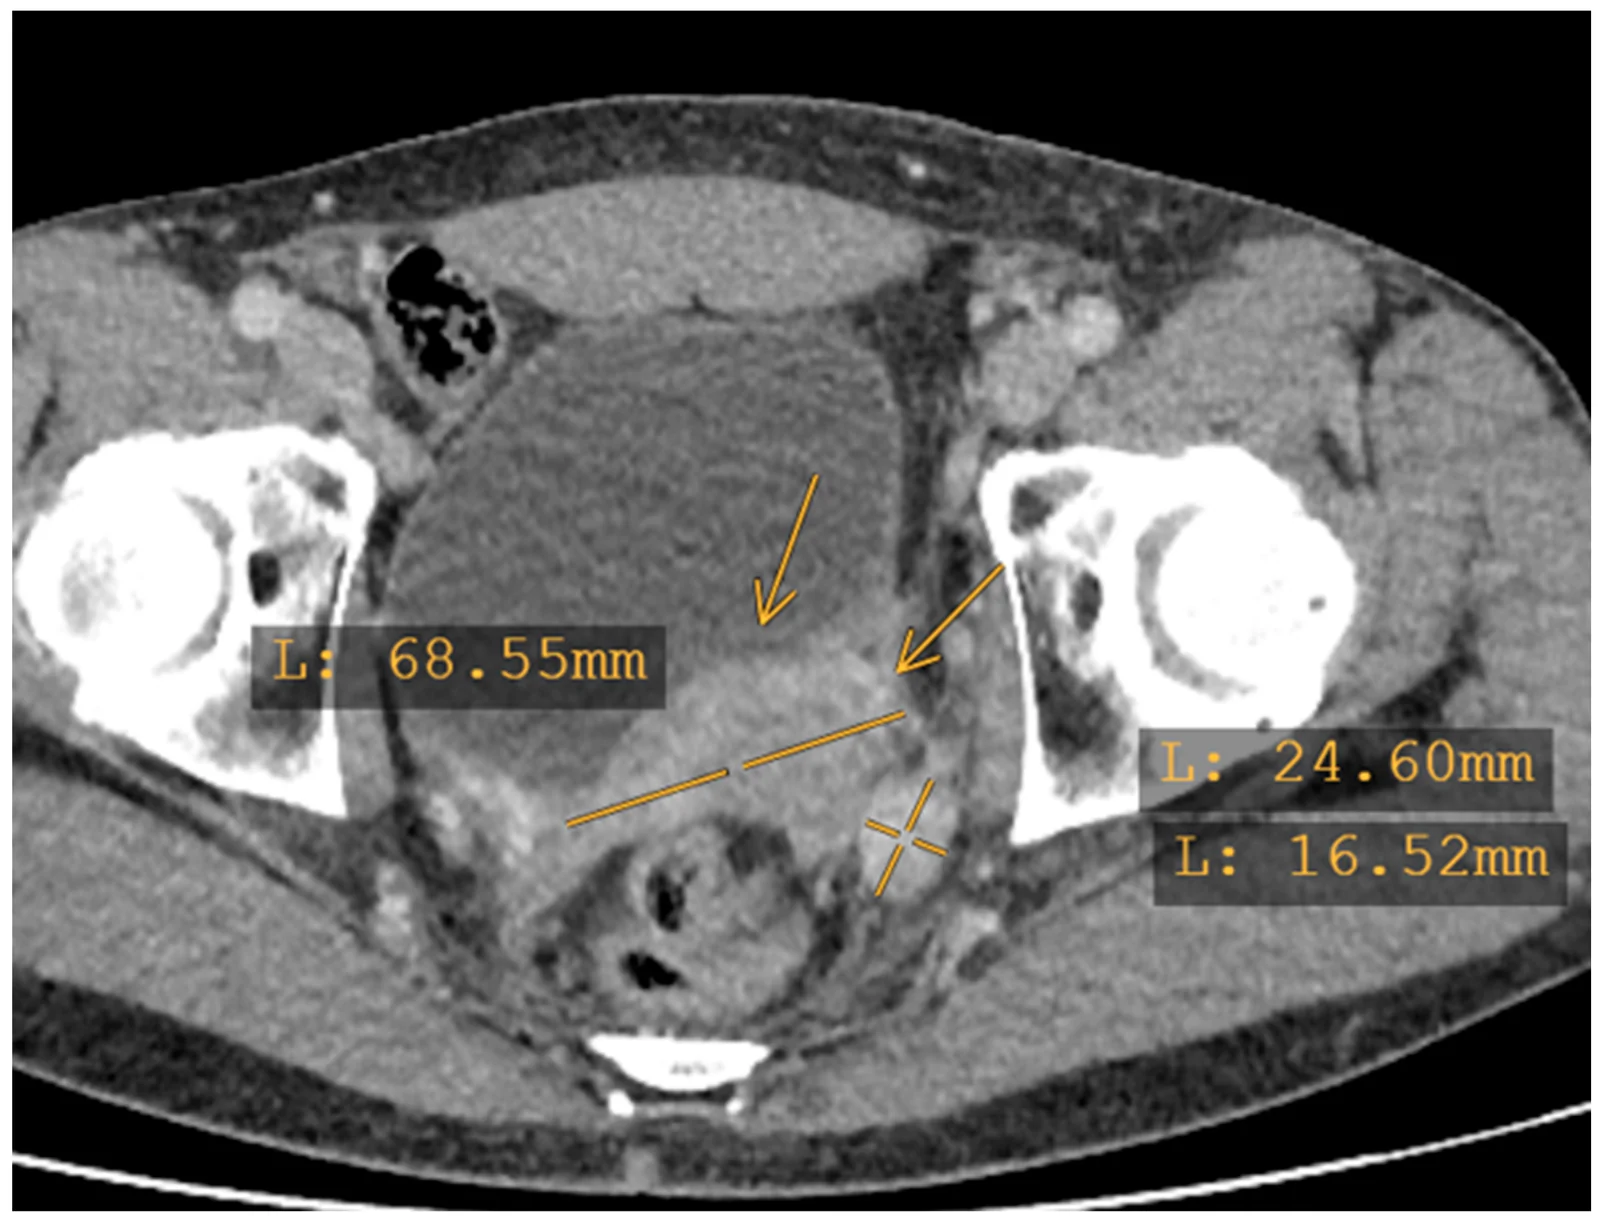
Baseline CT (03 April 2025) showing bilateral, locally advanced, high-grade prostate cancer obliterating the left rectoprostatic angle and infiltrating into the bladder floor (arrows); the image shows the base of the prostate (69 mm in diameter, represented by the diagonal line). A significantly enlarged local lymph node measuring 25 mm × 17 mm (length × width) is visible adjacent to the mesorectal fascia; on PSMA PET, this was confirmed as metastatic involvement. *Note.* The arrows indicate the areas of tumor extension, the measurement lines indicate the corresponding measurements, and “L” denotes the length of the respective measurement.
